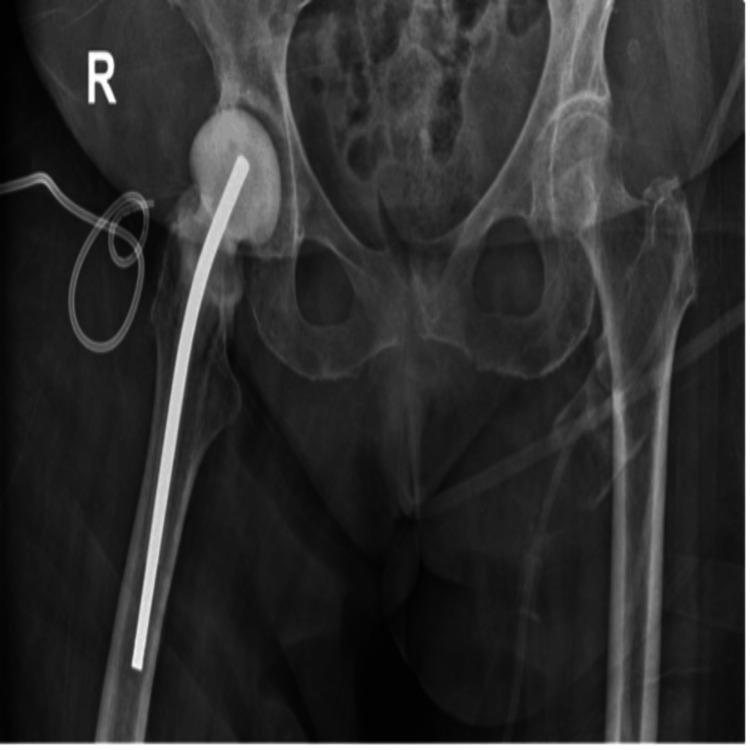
Plain radiograph during spacer interval
